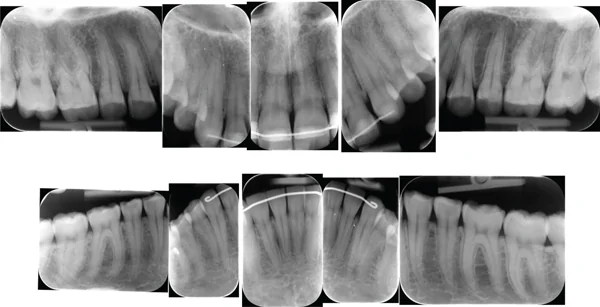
Baseline radiographic assessment.
